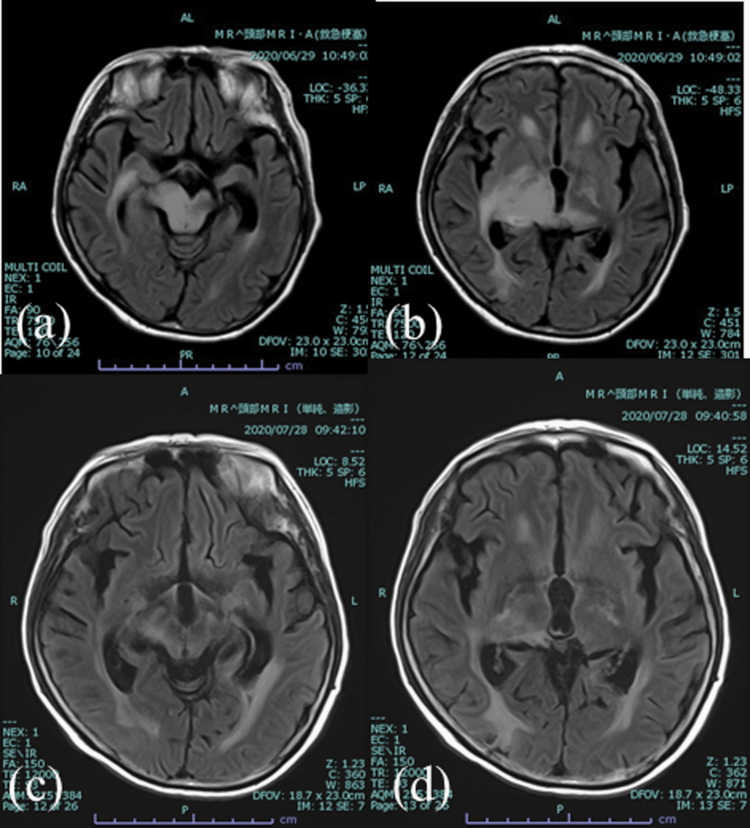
FLAIR image on admission (a,b) and 1 month after admission (c,d). The massive hyperintensity in the right thalamus and brainstem diminished significantly 1 month after treatment (c,d).
